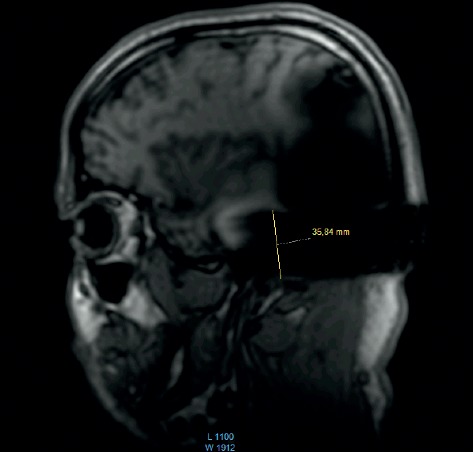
MRI survey pattern of the sagittal plain of a 1.5 T scanning of a Medel Synchrony implant.
